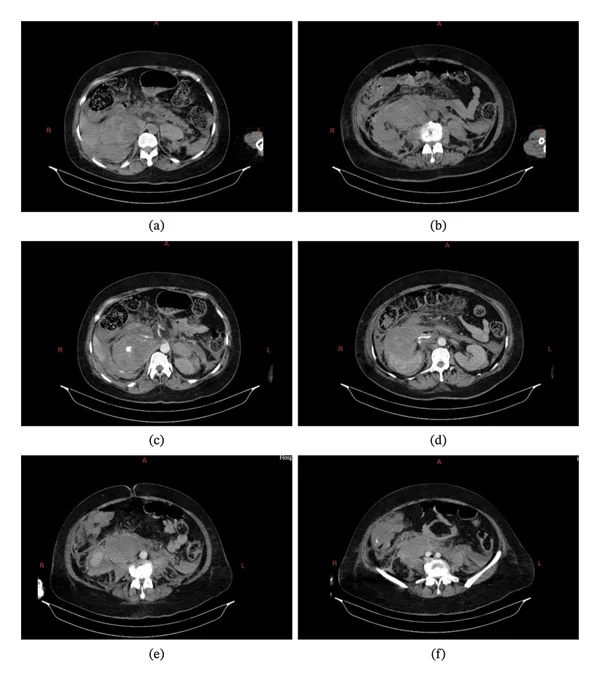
(a, b) Abdominopelvic CT scan shows a left adrenal mass and a massive hematoma of the right adrenal. (c–f) A left adrenal mass and a right adrenal hematoma with extravasation from intravascular to intrahematoma, presenting active bleeding.
